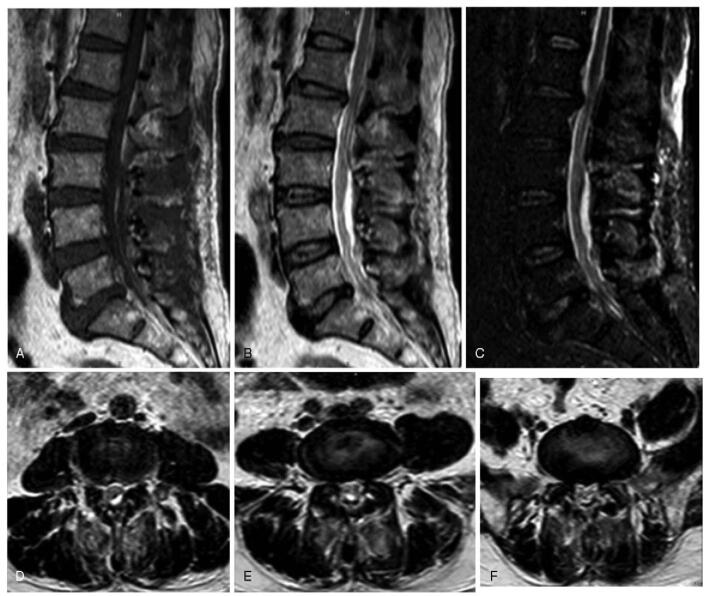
Postoperative MRI showed that the epidural fat had been removed and the dural sac compression was relieved. (A) T1-weighted sagittal image, (B) T2-weighted sagittal image, (C) fat-suppressed T2-weighted image, (D) lumbar axial MRI (L3–L4 level), (E) lumbar axial MRI (L4–L5 level), and (F) lumbar axial MRI (L5–S1 level). MRI = magnetic resonance imaging.
